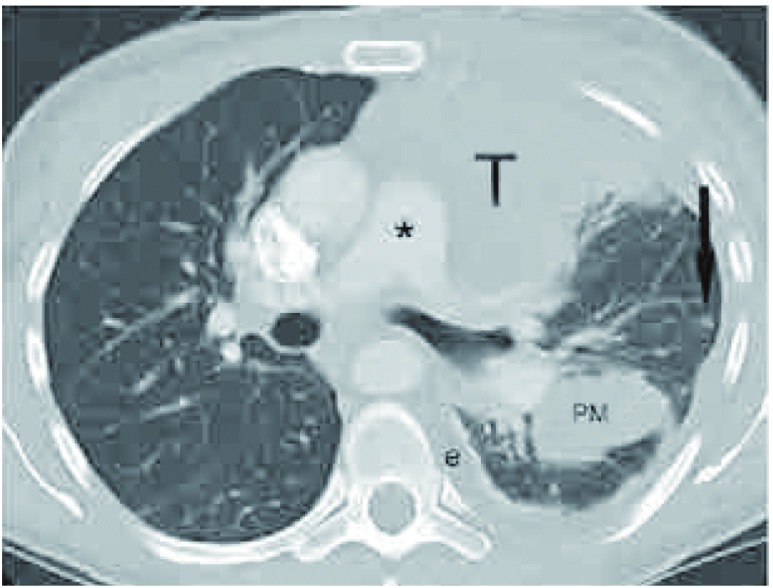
胸膜和肺转移。胸部增强CT（肺窗）显示，胸腺瘤紧邻肺动脉干（*）。左侧叶间裂胸膜转移（PM），左侧胸腔积液（e），和肺实质结节（黑箭头所示）。 Pleural and ulmonary metastasis. Contrast-enhanced chest computed tomography (CT) (lung window)demonstrates the thymoma (T) abutting the pulmonary trunk (*). There is a pleural metastasis within the left major fissure (PM), a left pleural effusion (e), and a pulmonary nodule (black arrow) surrounded by lung.
